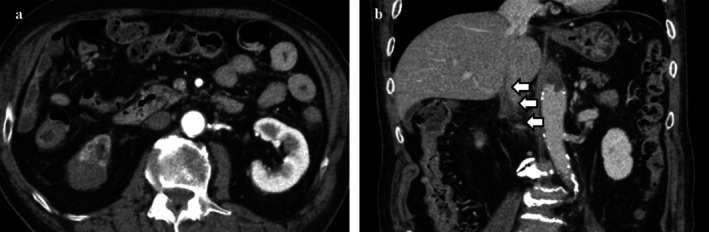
Preoperative contrast‐enhanced abdominal CT after 21 months of nivolumab plus ipilimumab therapy. Preoperative contrast‐enhanced CT performed 21 months after treatment initiation showed a reduction in renal tumor size to 29 mm (68.1% decrease from baseline) and regression of the IVC‐TT to 15 mm above the renal vein (Neves classification: Level 3 to level 1). (a) Axial view, corticomedullary phase; (b) Coronal view, corticomedullary phase. White arrows indicate the thrombus within the IVC.
